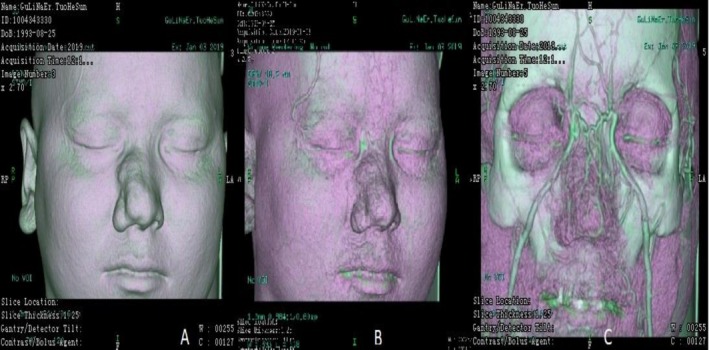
Contrast‐enhanced computed tomography with three‐dimensional vascular reconstruction of the nasal region. (A) Three‐dimensional surface reconstruction demonstrating the anatomical contour of the nasal region. (B) Vascular reconstruction revealing abnormal tortuous vascular channels in the nasal dorsum. (C) Enhanced vascular visualization showing prominent feeding vessels and abnormal arteriovenous connections consistent with an arteriovenous malformation.
